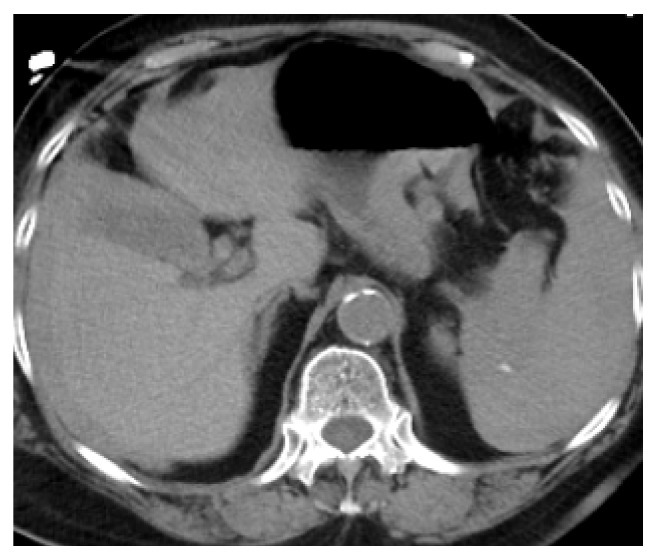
CT abdomen and pelvis without contrast showing hypodense area of bleeding.
